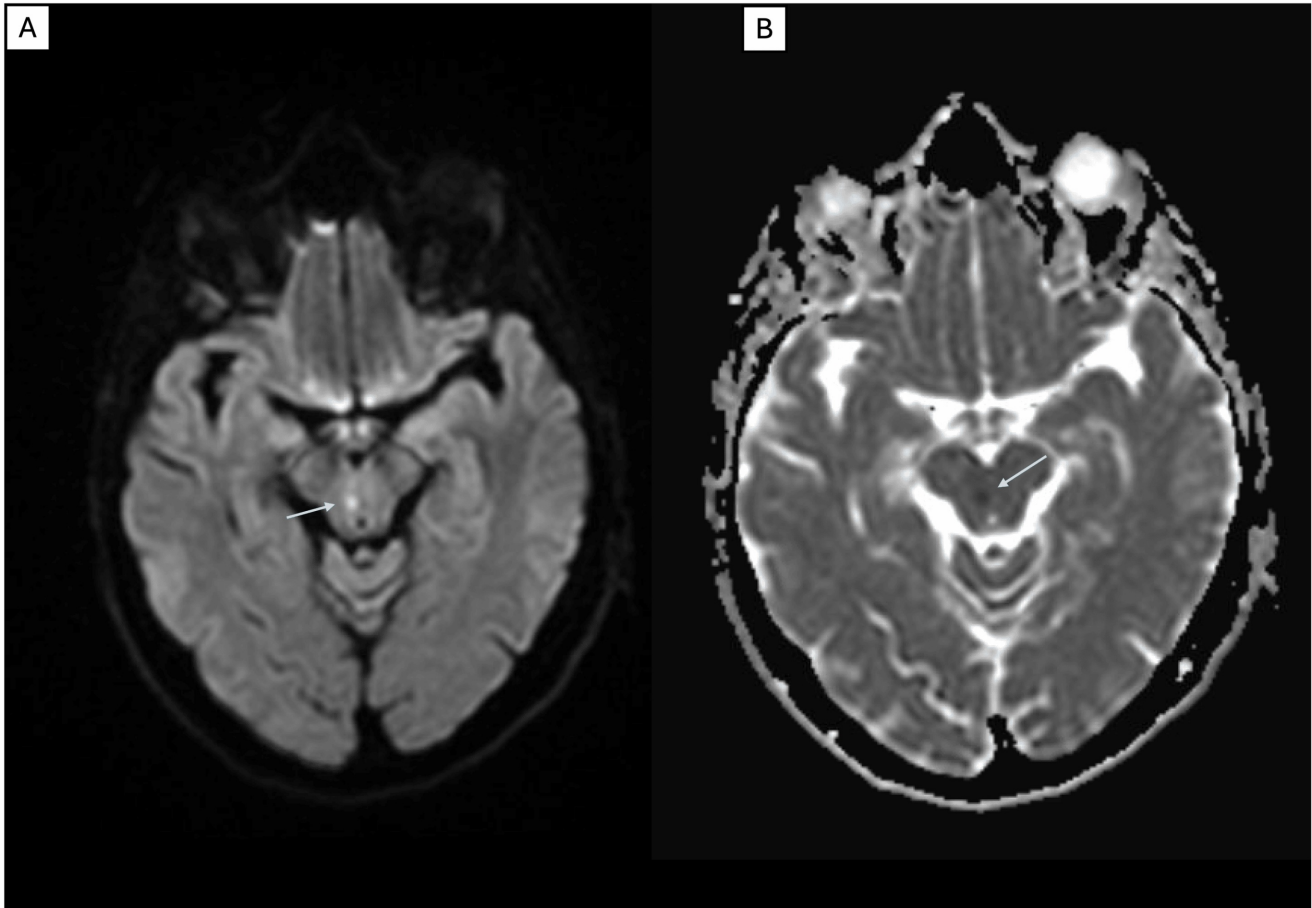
Right paramedian midbrain stroke demonstrated by MRI of the brain Axial images at the level of the midbrain. (A) Diffusion-weighted imaging (DWI) sequence. (B) Apparent diffusion coefficient (ADC) sequence. Restricted diffusion is evident in the right paramedian midbrain region on both DWI and ADC sequences, consistent with an acute lacunar midbrain stroke (arrows).
